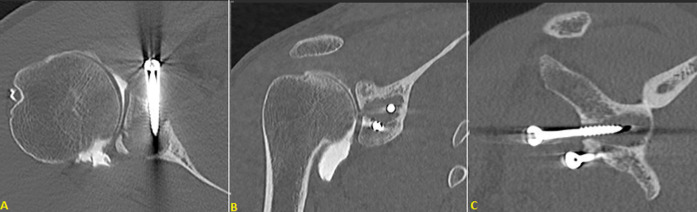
(A,B,C) CT scan of right shoulder; axial, coronal and sagittal slices, joint communication
